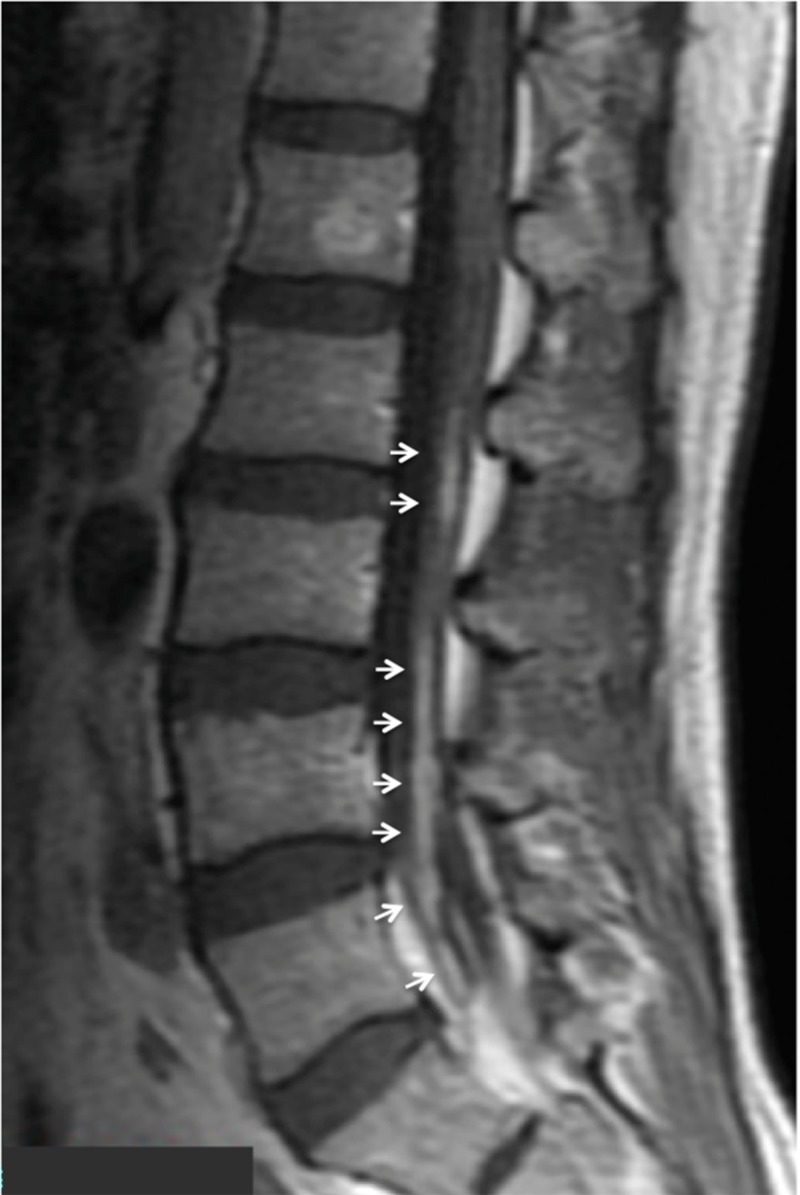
A sagittal view of lumbar magnetic resonance imaging (MRI) that disclosed gadolinium-enhancement in the cauda equina (white arrows).
